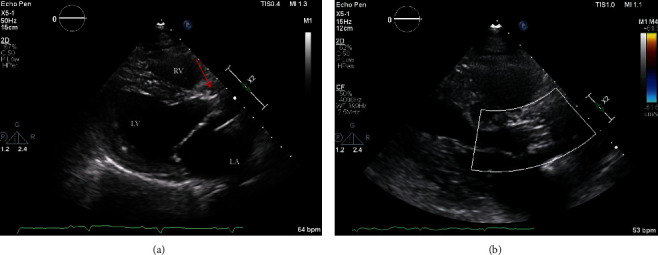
Transthoracic echocardiogram parasternal long-axis view. (a) Valve-in-valve TAVR (red arrow) with some artifacts causing suboptimal resolution and visualization of the bioprosthesis, yet no overt large vegetations were detected. No pericardial effusion present and systolic function was preserved (55%). (b) Diastolic frame showing no evidence of significant regurgitation by color Doppler. RV: right ventricle; LV: left ventricle; LA: left atrium.
